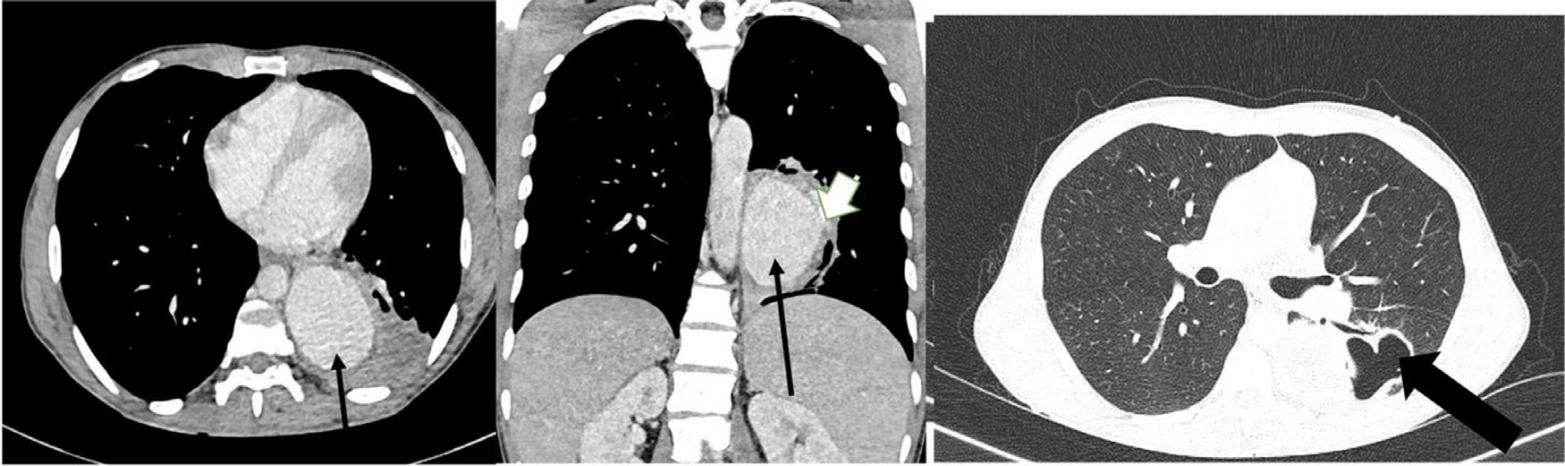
One-week post-treatment chest CT scan. Contrast enhanced chest CT scan shows a saccular aneurysmal dilation (straight arrow in A and B) of the left lower lobe segmental pulmonary artery (short broad arrow in B) measuring 7.0 (TRV) x 5.8 (AP) x 7.0 (CC) cm with an adjacent irregular cavitary lesion (long broad arrow in C).
